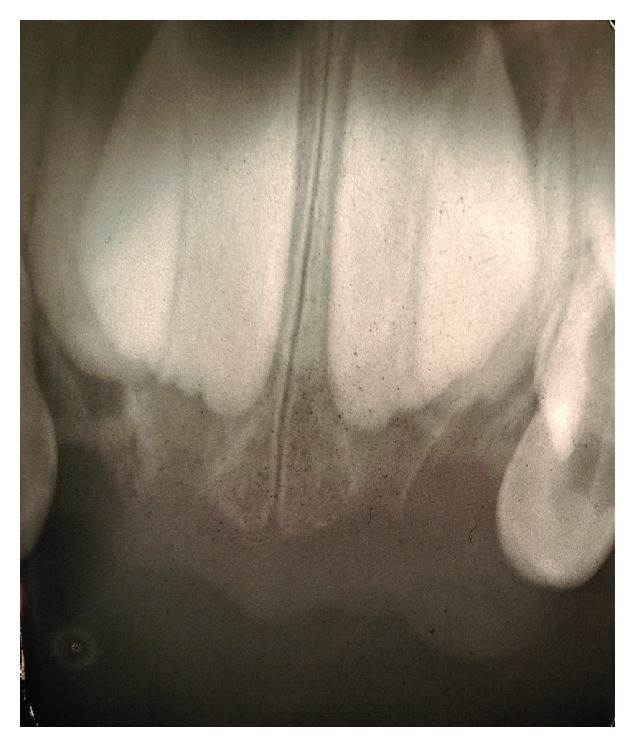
Intraoral periapical radiograph, showing superficial erosion of the alveolar bone.
